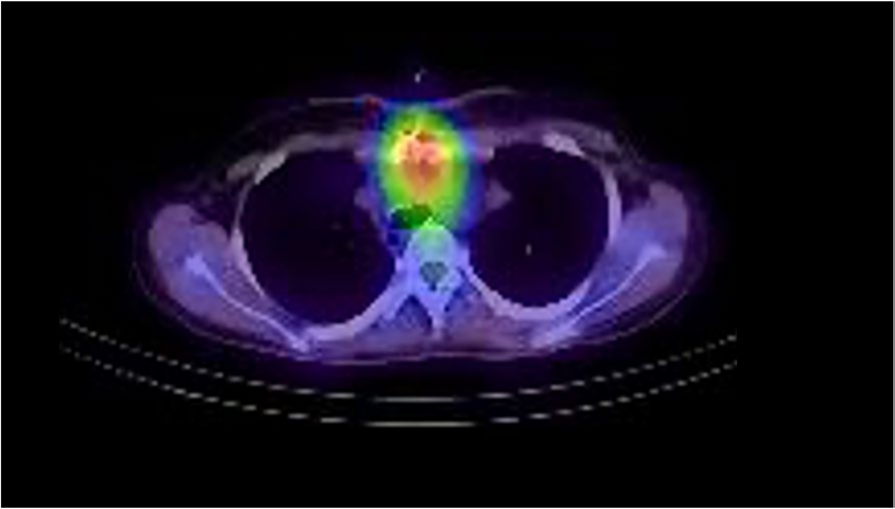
Gallium scintigraphy result
